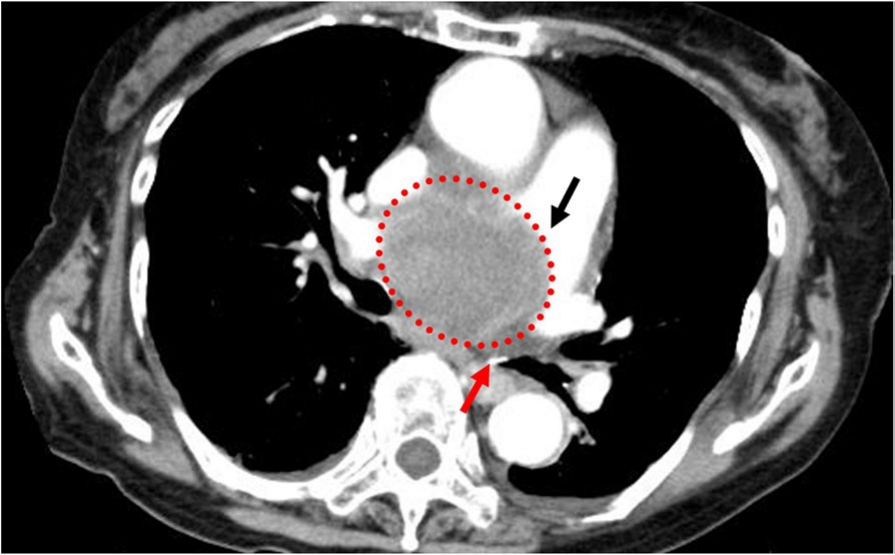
Preoperative CT scan showing a large thoracic aortic aneurysm (TAA) (red dotted line circle) impending rupture. TAA is compressing the pulmonary artery (black arrow) and left bronchi (red arrow)
